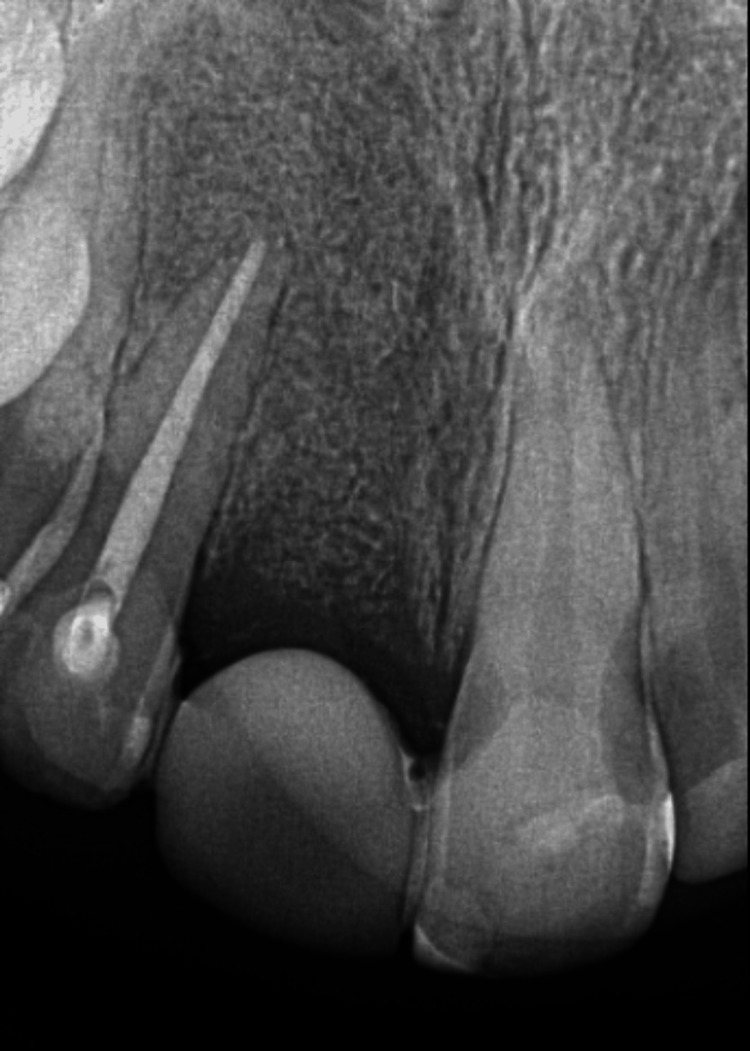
Three-month follow-up. Periapical radiograph showing no evidence of periapical radiolucency and signs of bone healing.
